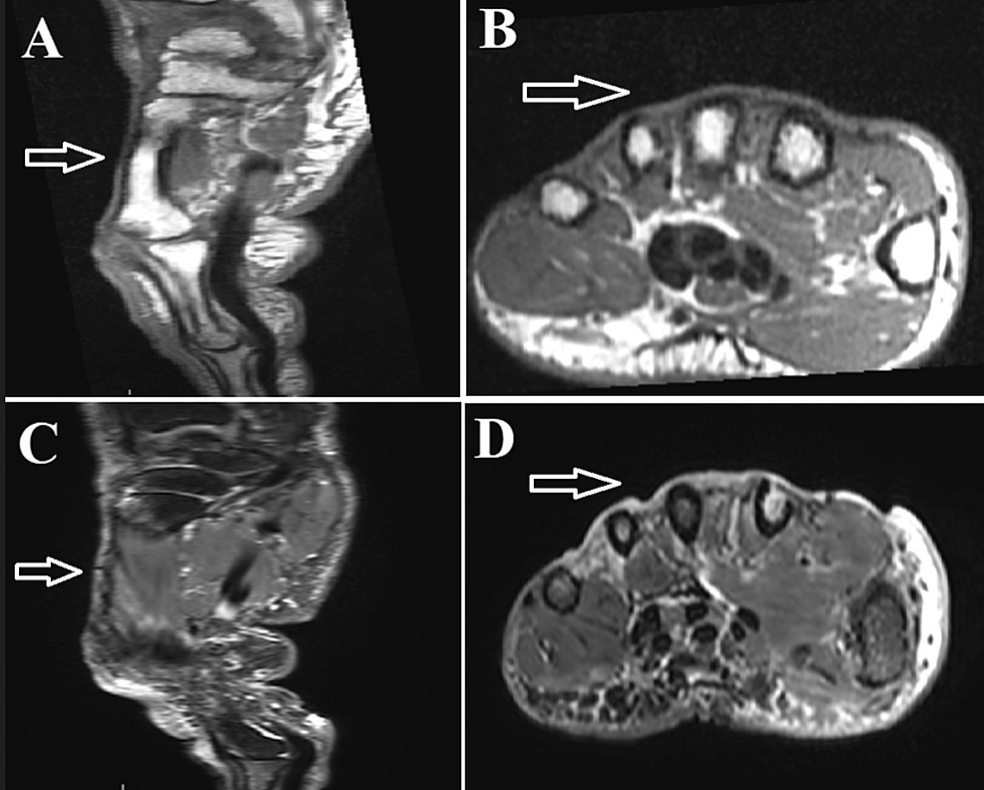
Magnetic resonance imaging (MRI) of the right hand at one-year follow-up showing no recurrence at the site of initial lesion (white arrows). (A) Sagittal T1-weighted MRI image, (B) Axial T1-weighted MRI image, (C) Sagittal fat-suppressed T2-weighted image, (D) Axial fat-suppressed T2-weighted image.
